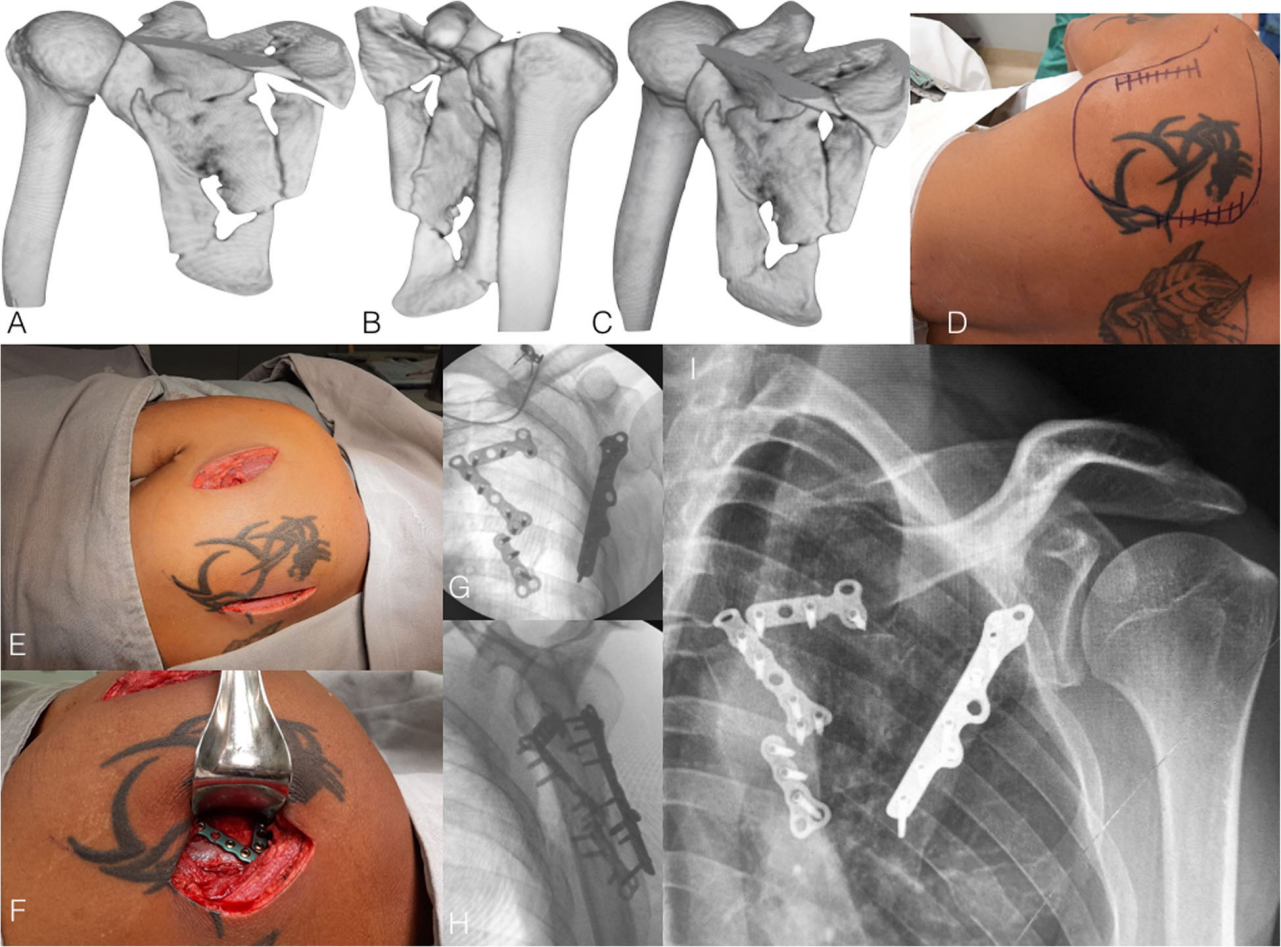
a, b, and c: 3-D CT reconstruction showing a comminuted infraglenoid fracture of the scapular body in a 35-year-old male patient. Observe the angulation of the inferior part of the scapular body and the medialization of the glenoid. d: Preoperative photography depicting the landmarks for minimally invasive approach. e and f: Perioperative photographs showing the lateral (between infraspinatus and teres minor muscles) and medial approaches (partial detachment of the infraspinatus). g and h: Postoperative fluoroscopy images in anteroposterior and lateral views showing fracture reduction and fixation using 2.7 minifragment plates (medial pillar) and the unconventional use of a 2.7 fibular plate (lateral pillar)
